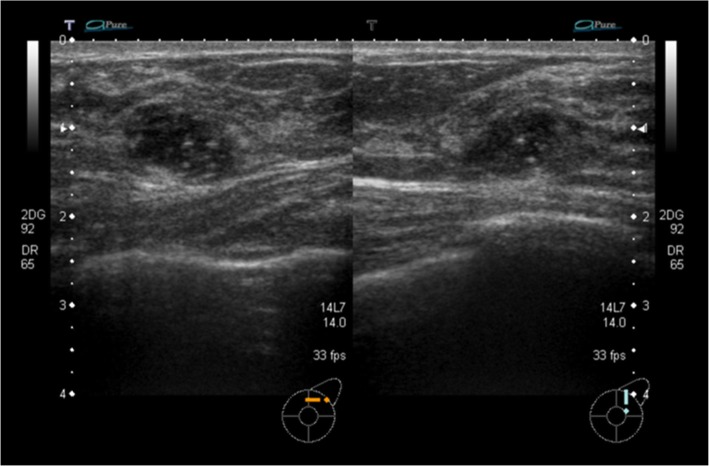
Ultrasonography. Ultrasonography showing a hypoechoic irregular mass (13 mm × 12 mm × 7 mm) with clear roughening of the boundary and a high echo spot inside in the left CD area
